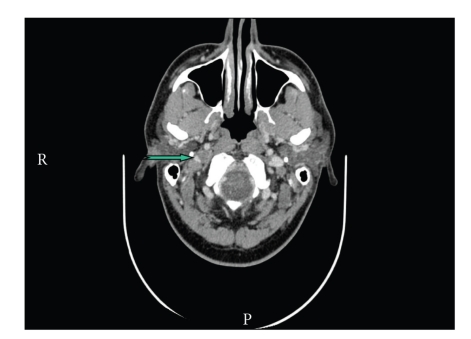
CT neck with contrast at three-month followup showing that a focal filling defect consistent with thrombus is noted in the distal sigmoid sinus on the right side extending into the right internal jugular vein up to the level of C2 vertebra. There is partial recanalisation of the previously thrombosed segment of the right internal jugular vein (notched arrow). Normal appearances of the internal carotid artery and internal jugular vein on the left side are shown.
